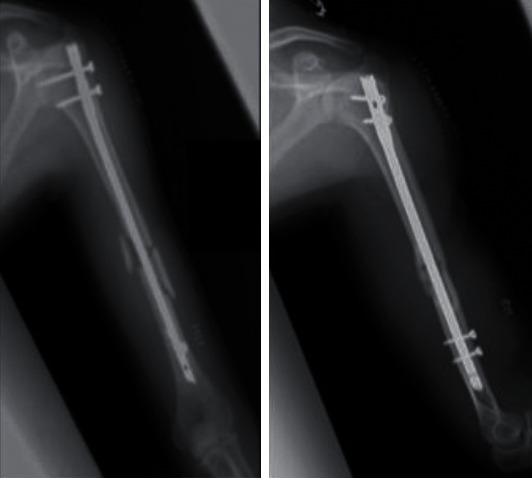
Radiographs just after the first surgery using IMN. The surgery was performed using a curved nail 8 days after the injury.
